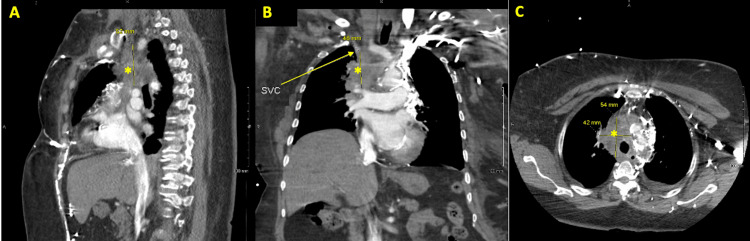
Chest computed tomography. Chest computed tomography (CT) scan shows 54 mm lymphoid mass in the mediastinum (*, asterisk) sagittal view (A). On a coronal image, the mass (*) is extended to the right atrium and compressing the superior vena cava (SVC, arrow) and right brachiocephalic vein (arrow) (B). Transverse lung window CT image shows the mass (*, 54 mm × 42 mm) fully occupying and compressing the SVC (not shown) (C). The vessels on the left side of the mediastinum are opacified, likely related to SVC obstruction.
